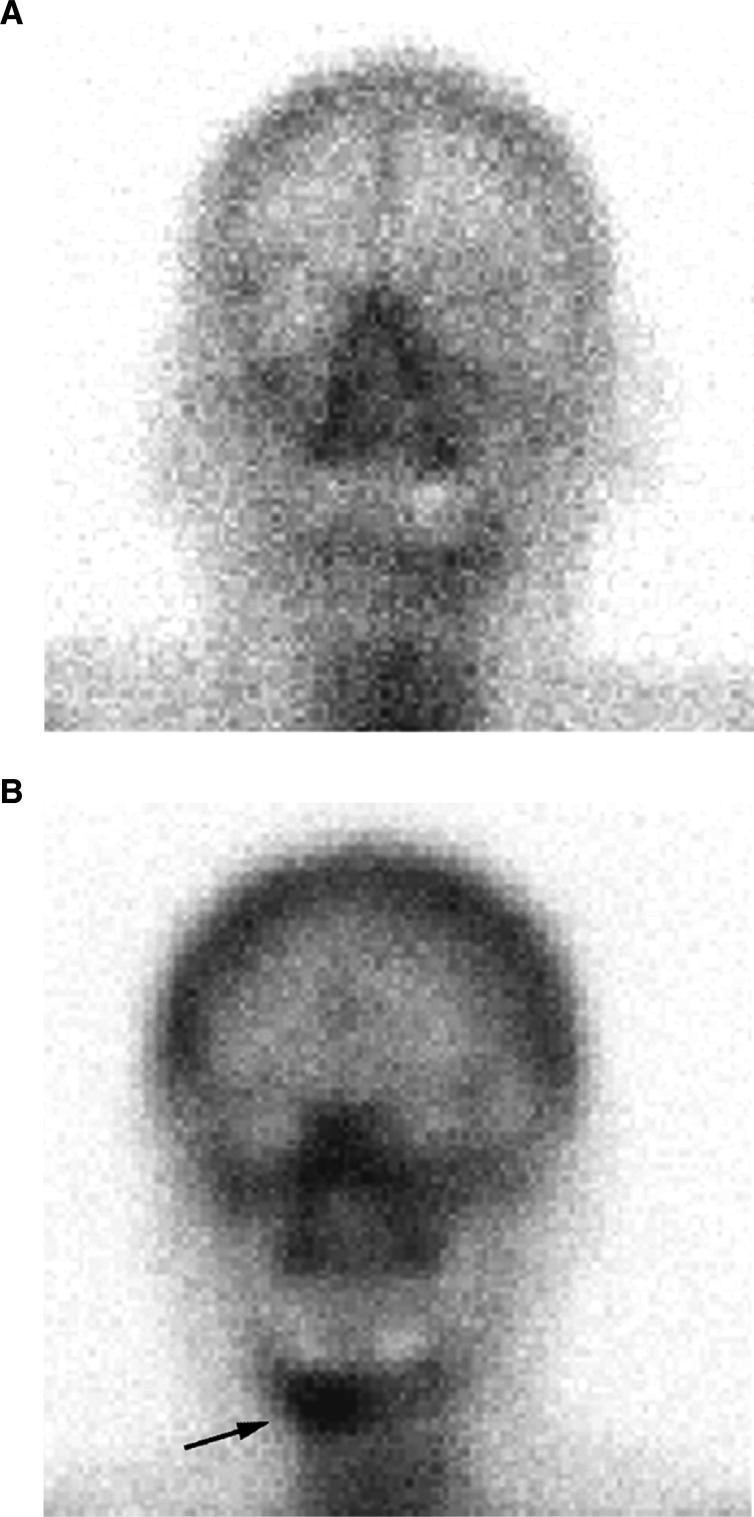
(A,B) Scintigraphy of a patient with an OSCC with (A) and without (B) infiltration of the mandibular bone. Technetium 99m MDP bone scans with planar imaging and SPECT were performed in the course of the staging. No increased activity is visible in (A), but a lesion of the right mandible is seen in (B) (arrow). For the chromatograms of these patients see Figure 3A and B.
